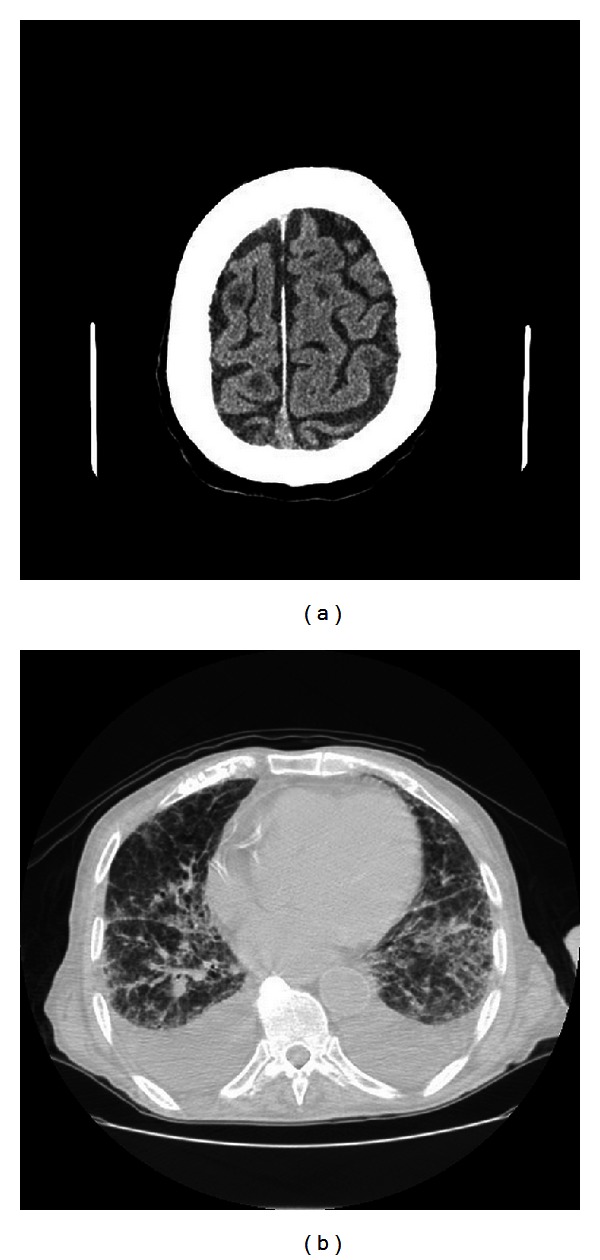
(a) Computerized tomography (CT) scan of the patient's brain showing multiple enhancing focal lesions. (b) Chest CT scan showing multiple nodular lesions and bilateral pleural effusion.
